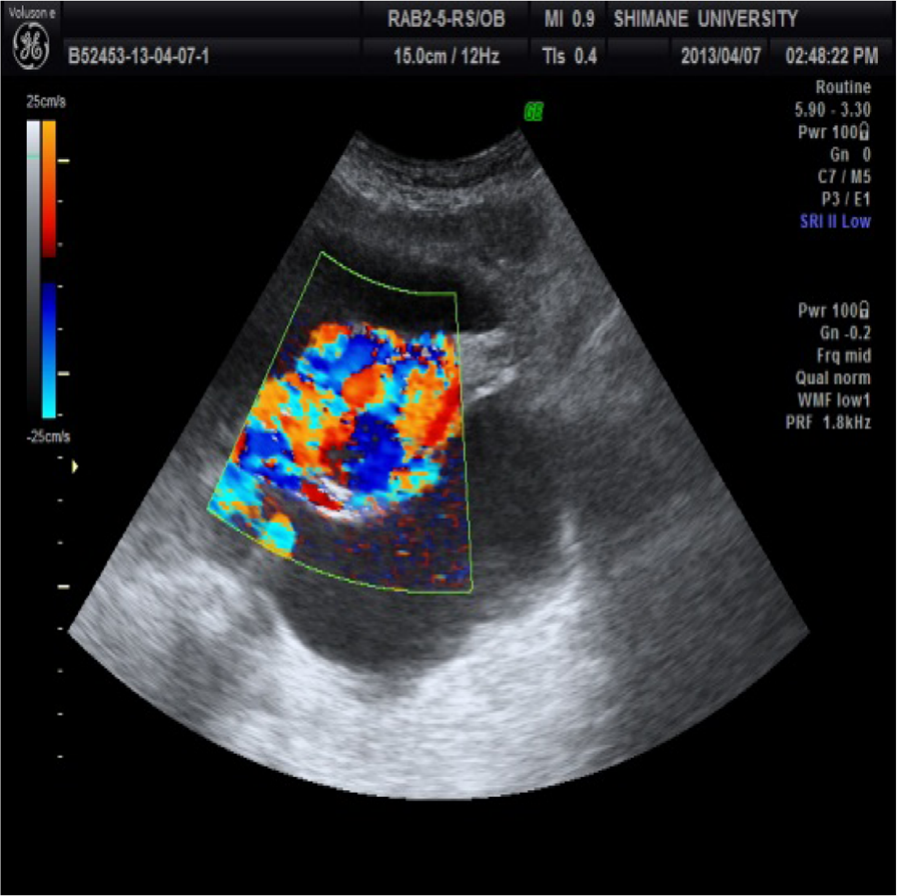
Color flow Doppler ultrasound shows a mosaic pattern of blood flow within cystic spaces in the uterine body.
